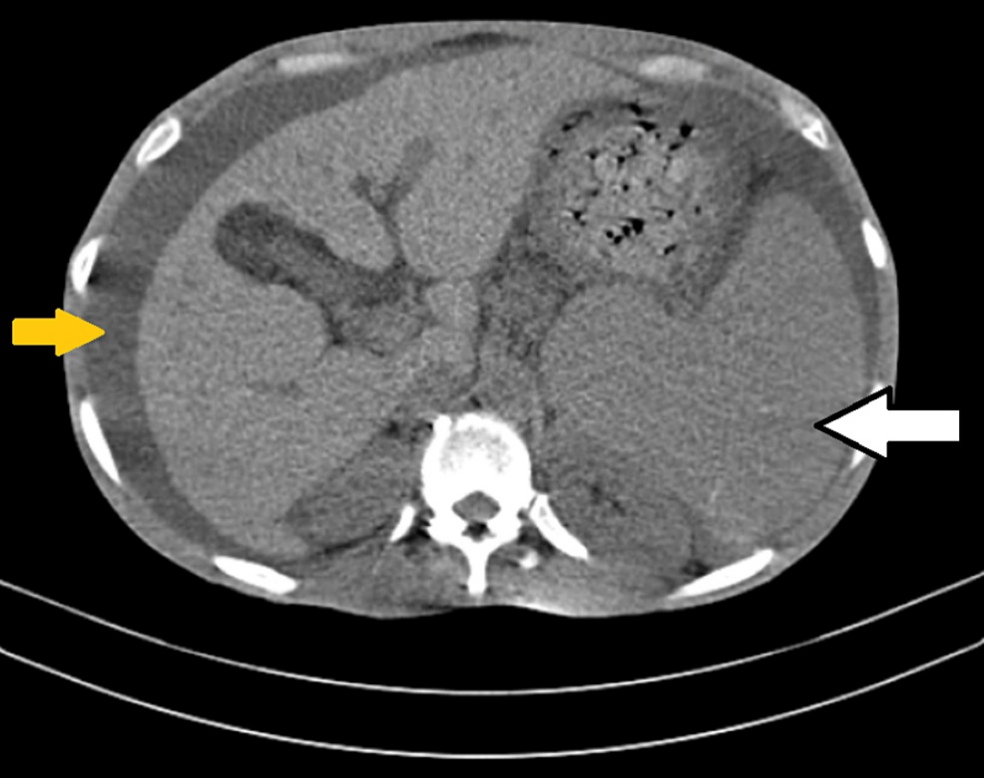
Computed tomography of the abdomen with a white arrow showing splenomegaly and a yellow arrow showing ascites
